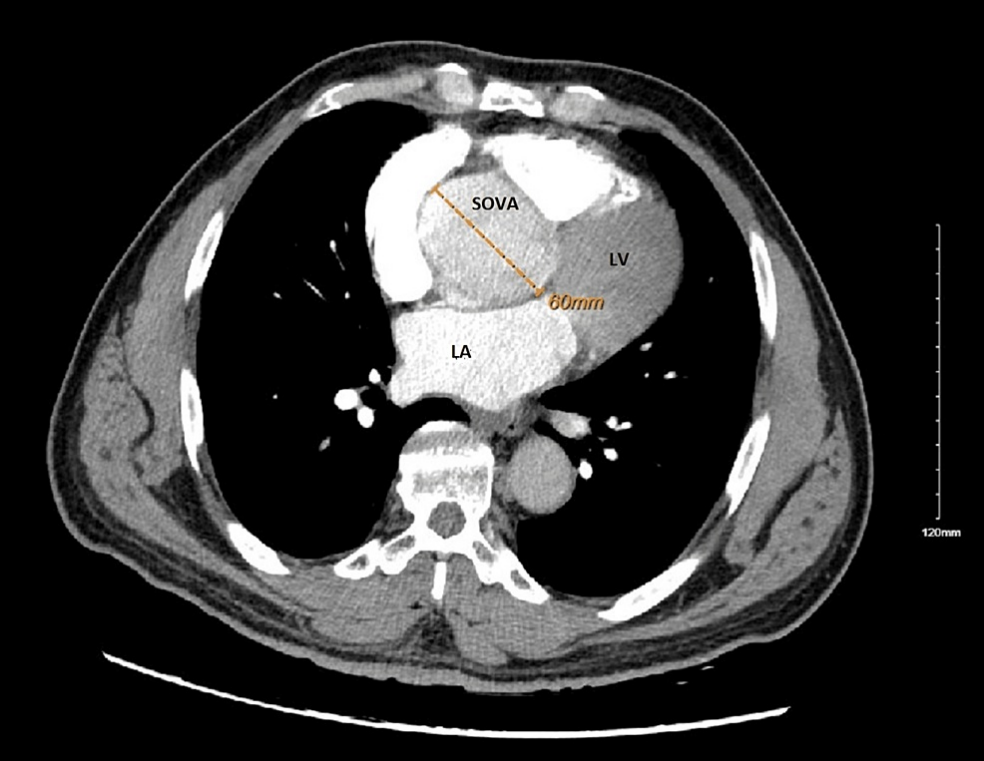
CT chest revealing 6 cm dilated sinus of Valsalva aneurysm LA: Left Atrium; LV: Left Ventricle; SOVA: SInus of Valsalva Aneurysm
